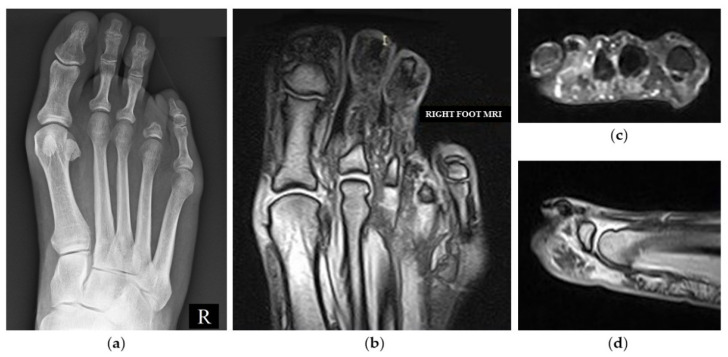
Weightbearing X-ray AP image of the right foot showing the fourth toe amputation at the level of the base of the P1 after surgery (a). MRI of the right forefoot performed at 6 months from the amputation, showing flattening of the plantar arch, signs of overload of the fourth metatarsal head and persistence of suprafascial plantar edema (b) coronal; (c) axial; (d) sagittal view.
